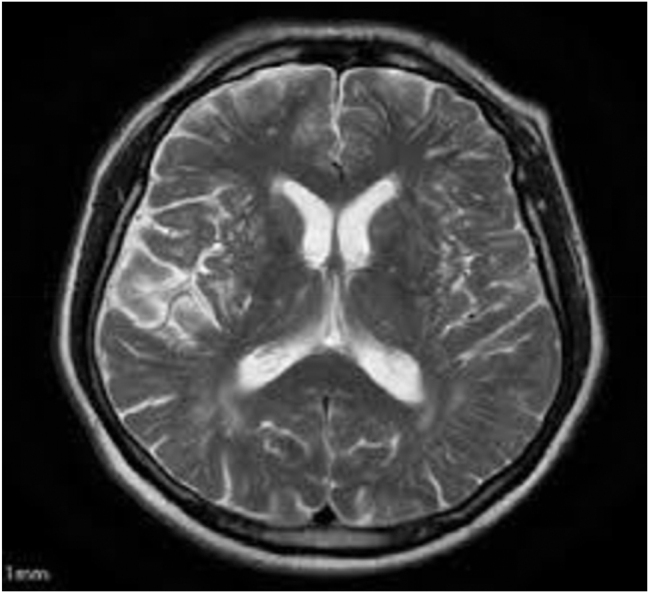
Magnetic resonance imaging (MRI) of the endocranium.
